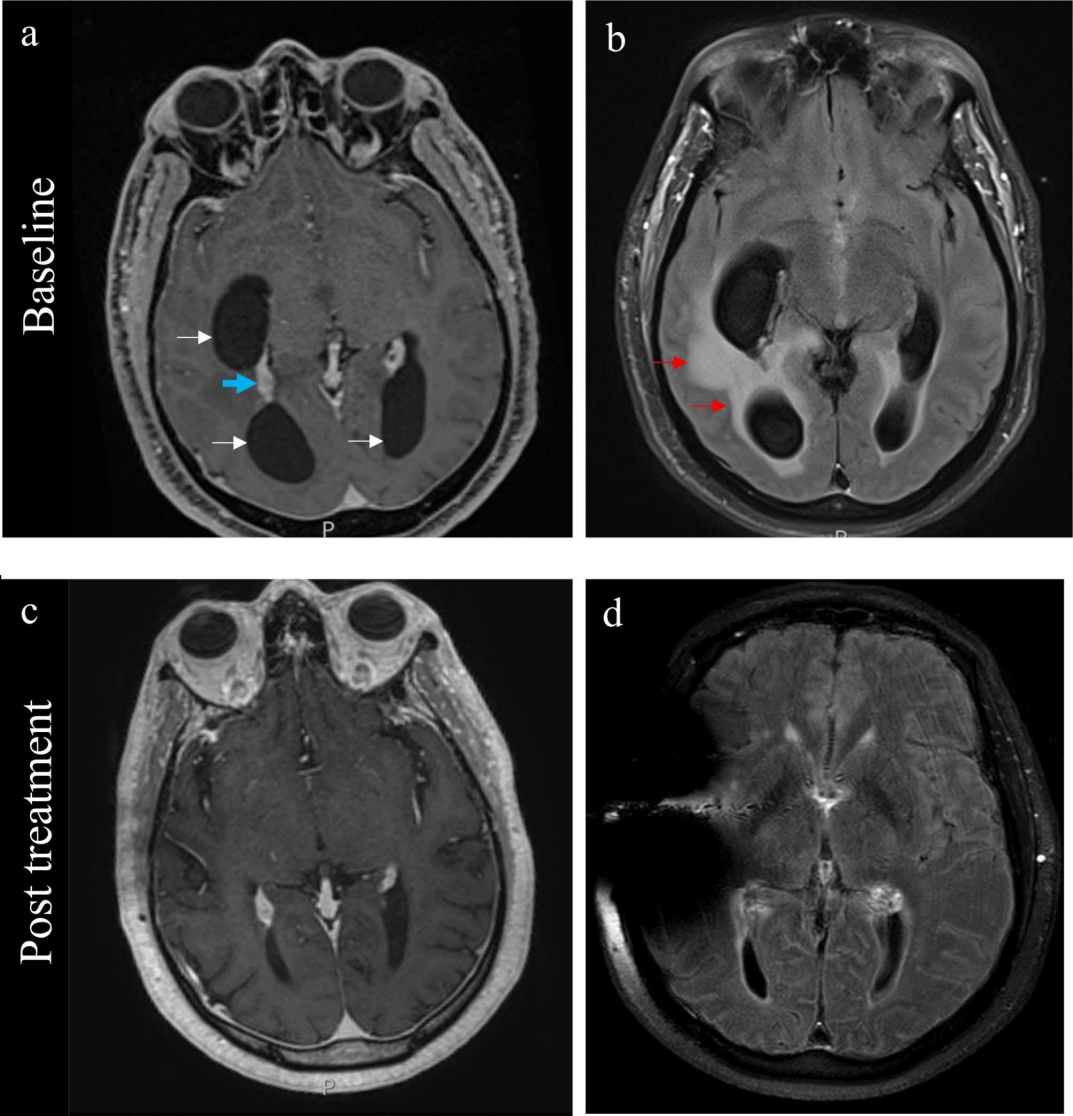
MRI scans of the brain before and after corticosteroid treatment: (a) Post-contrast T1-weighted imaging showing significant ventricular enlargement (white arrows) with enhancing choroid plexi (blue arrow) consistent with choroid plexitis. The occipital and temporal horns of the lateral ventricles are entrapped likely due to inflammatory adhesions. (b) Post-contrast FLAIR imaging showing periventricular edema consistent with transependymal CSF seepage associated with the enlarged and entrapped ventricles (red arrows). (c) Both choroid plexitis and entrapment significantly improved 60 days post steroid treatment with decreased periventricular transependymal CSF seepage (d)
